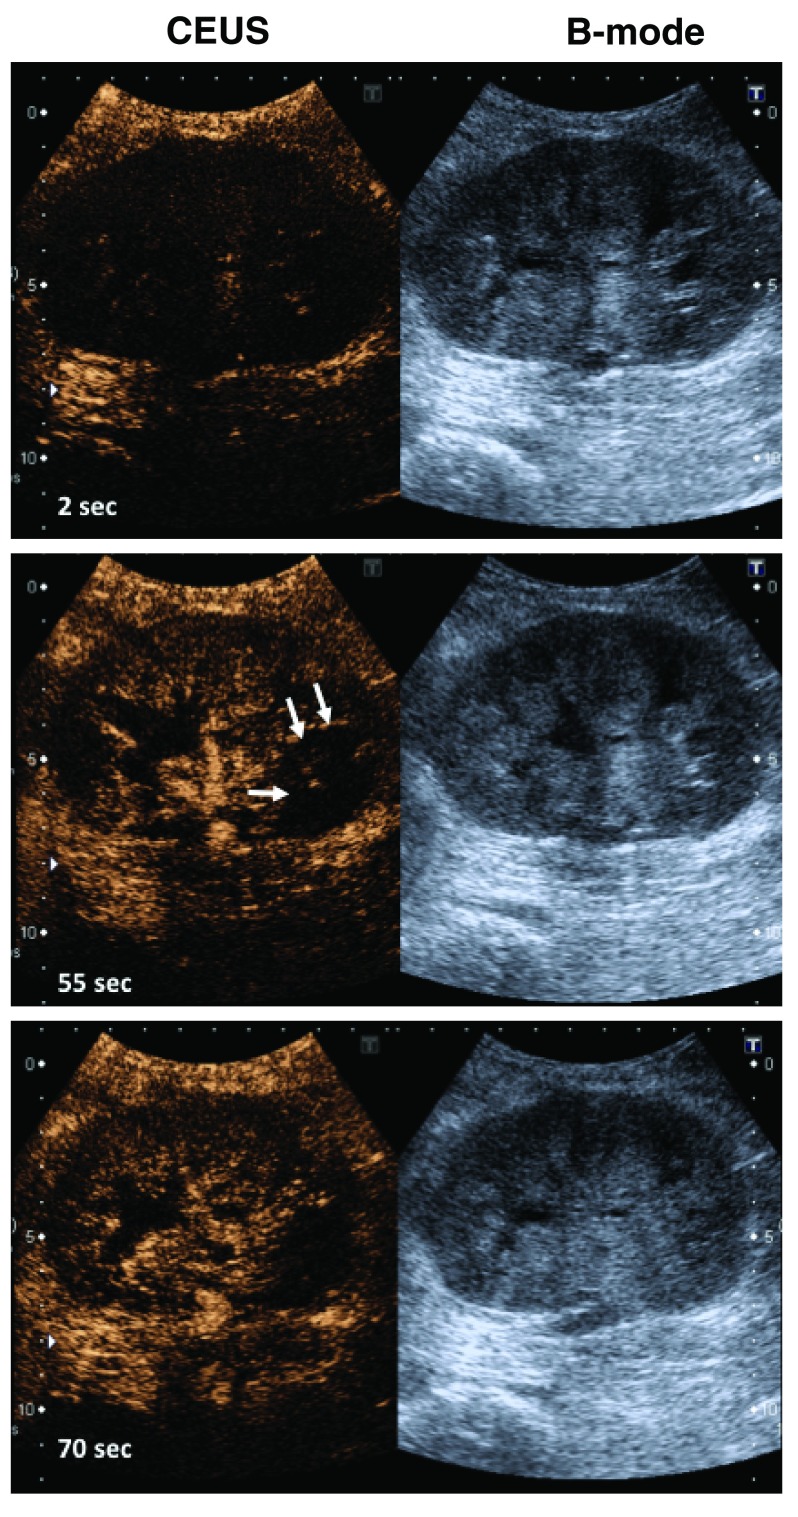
Contrast enhanced ultrasound (CEUS) and B-mode ultrasound of kidney allograft with acute rejection. The left panel shows the enrichment of the microbubbles within seconds in the renal vessels of the allograft of a patient with severe rejection. Areas in the lower pole of the kidney are hypoperfused after microbubble injection, indicating less blood flow to this region (arrows). The right panel corresponds to the B-mode ultrasound. These images are original, unpublished data (F. Gueler).
